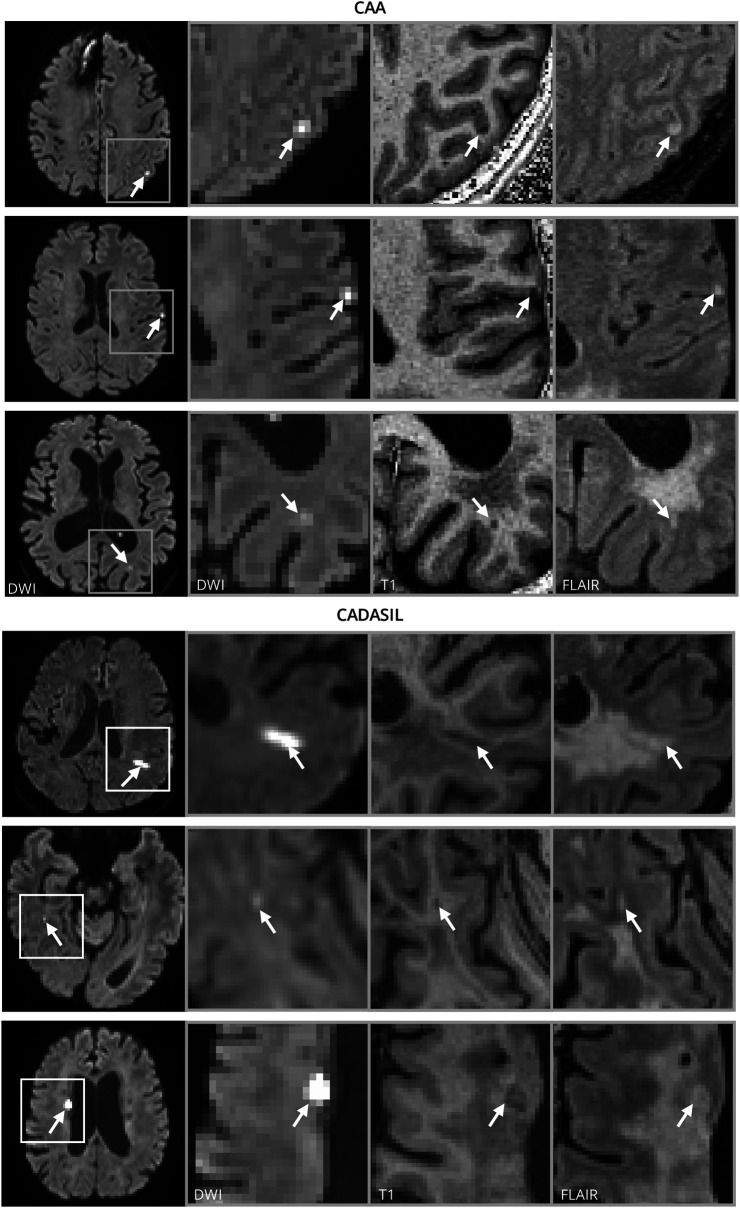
Examples of Incidental DWI-Positive Lesions in CAA and CADASIL on DWI (b = 1,000 s/mm^2^ trace), T1 and FLAIR CAA: shown are 2 cortical (top and middle row) and one white matter incidental DWI-positive lesions (bottom row). CADASIL: shown are 3 white matter incidental DWI-positive lesions. CAA = cerebral amyloid angiopathy; CADASIL = cerebral autosomal dominant arteriopathy with subcortical infarcts and leukoencephalopathy; DWI = diffusion-weighted imaging; FLAIR = fluid-attenuated inversion recovery.
